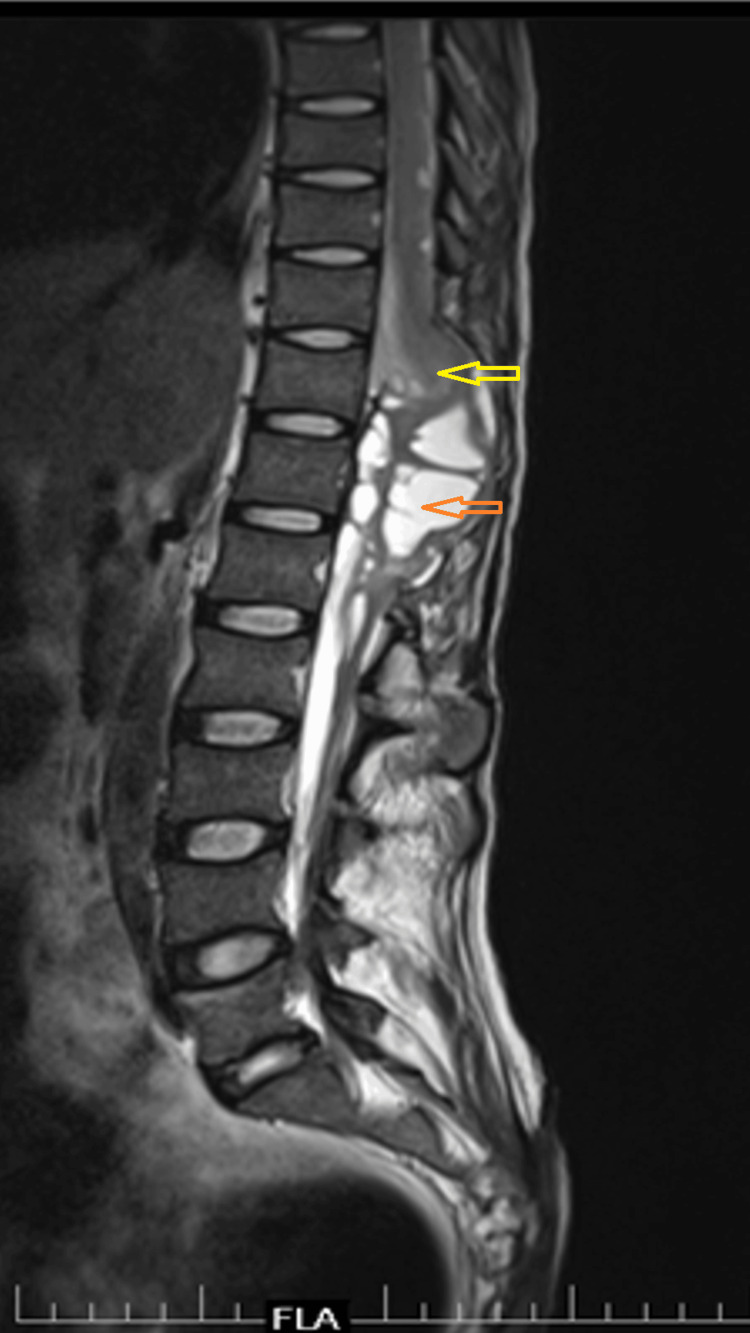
An MRI of the thoracolumbar spine (mid-sagittal section) Features of arachnoiditis, loculated cerebrospinal fluid (CSF) collection (orange arrow), and dorsal spinal cord herniation (yellow arrow) with effacement of all the neural tissue are seen.
